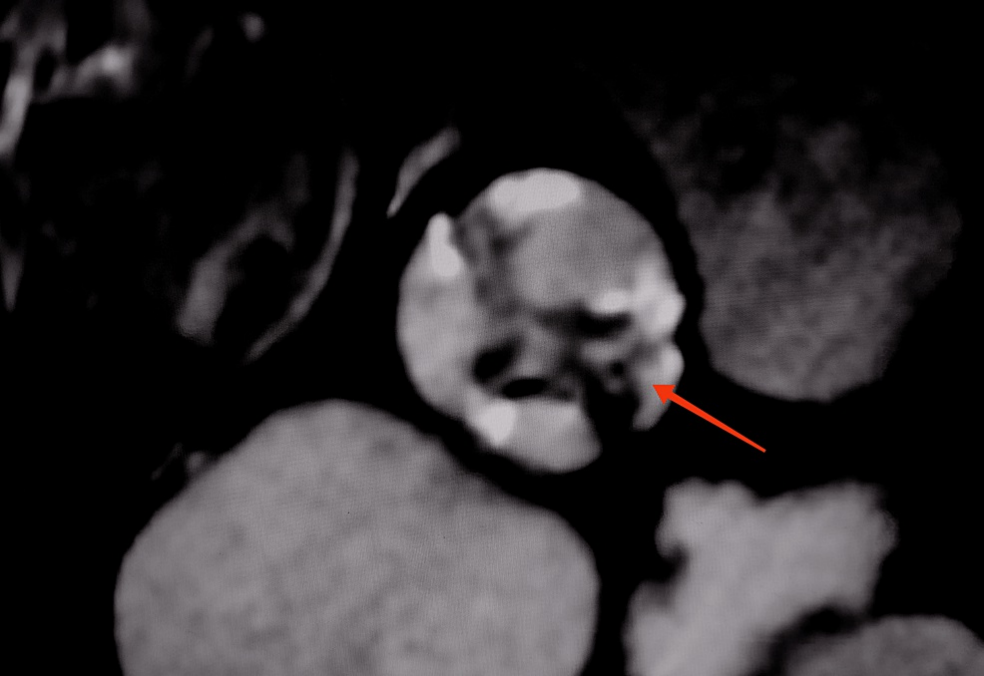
Multidetector computed tomography revealed a quadricuspid aortic valve with two equal-sized large cusps and two unequal smaller cusps (Red arrow).
